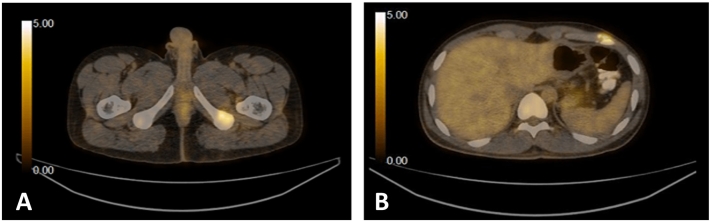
PET-CT demonstrating (A) stable FDG avidity over the left 6th rib and (B) improvement in FDG uptake over the left ischium.
